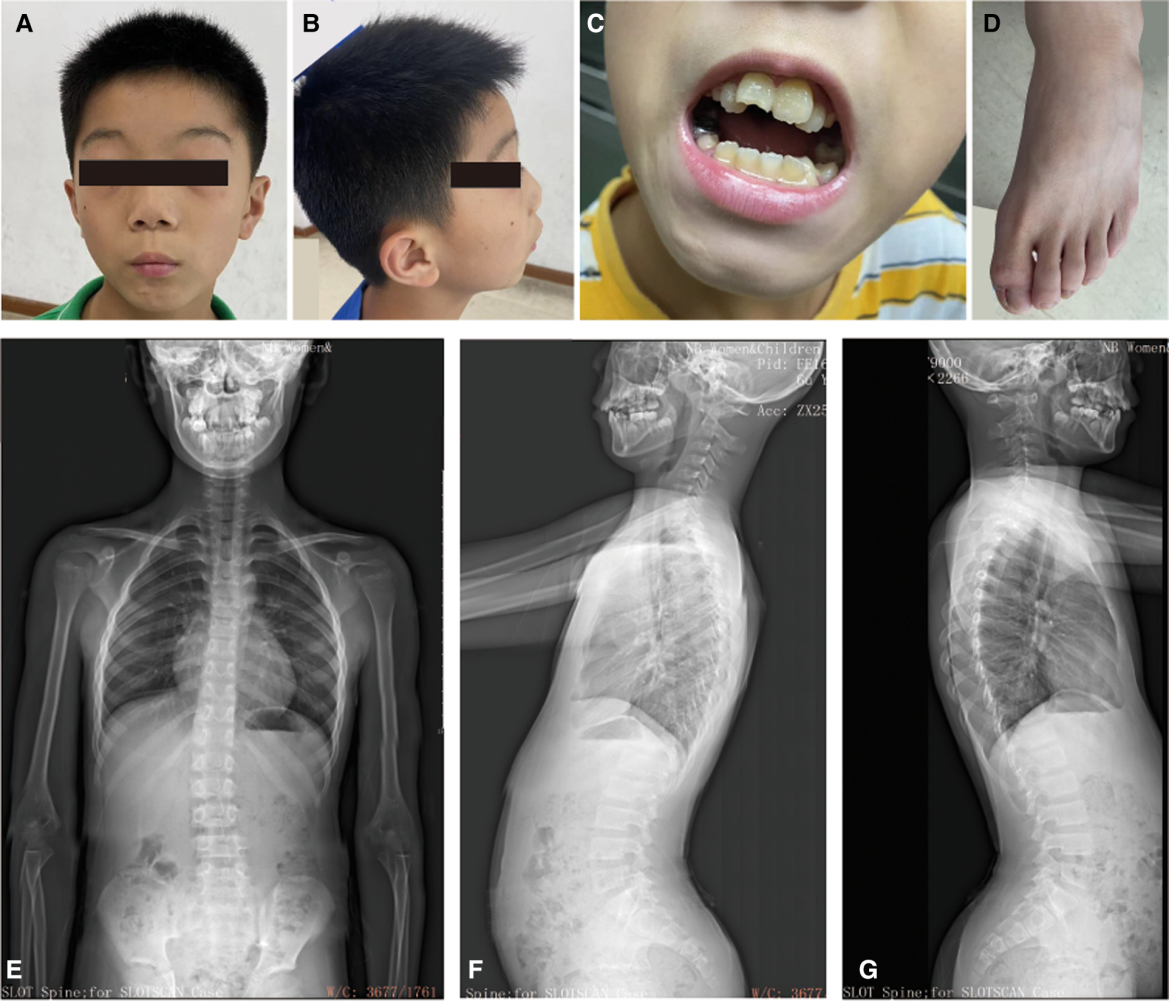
The clinical information for the patient. (A–C) Facial dysmorphology, dental malocclusion, and abnormalities in foot skin. (D) Bone age radiograph obtained at the age of 11 years (left hand). The Bone age corresponds to actual age. (E–G) Scoliosis x-rays showing a T12-L1 right curvature, Cobb angle of 11.5°. He has an anterior pelvic tilt, forward-leaning spine, increased thoracic kyphosis, and anterior cervical tilt.
